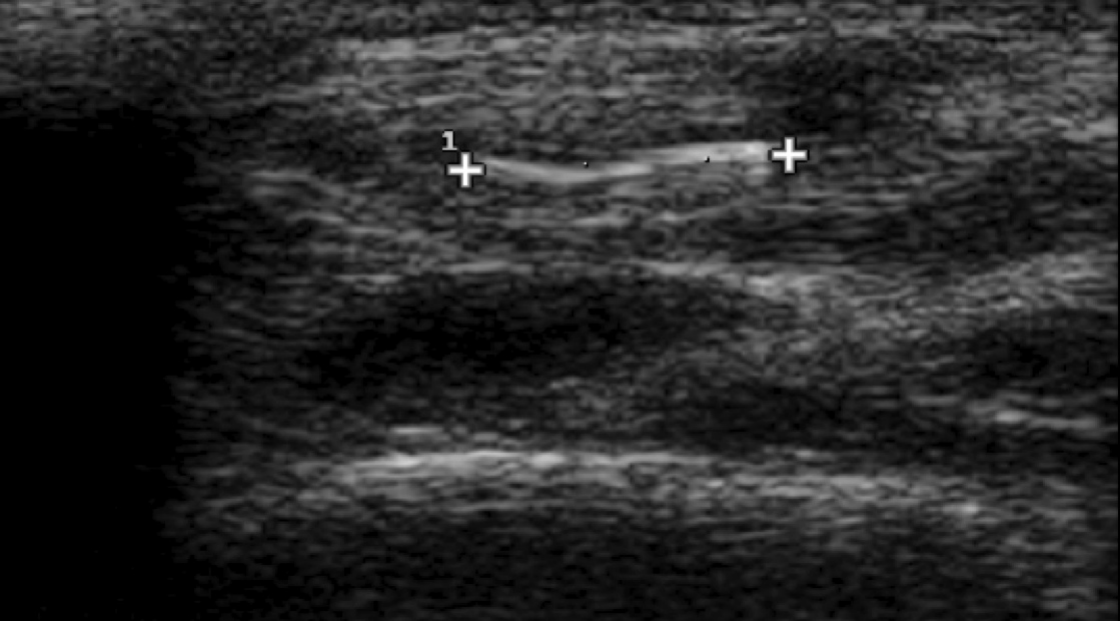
Longitudinal sonogram shows a hyperechoic 4 mm long broken glass (long arrow) without posterior acoustic shadowing in the forearm of a 24 years old man.
